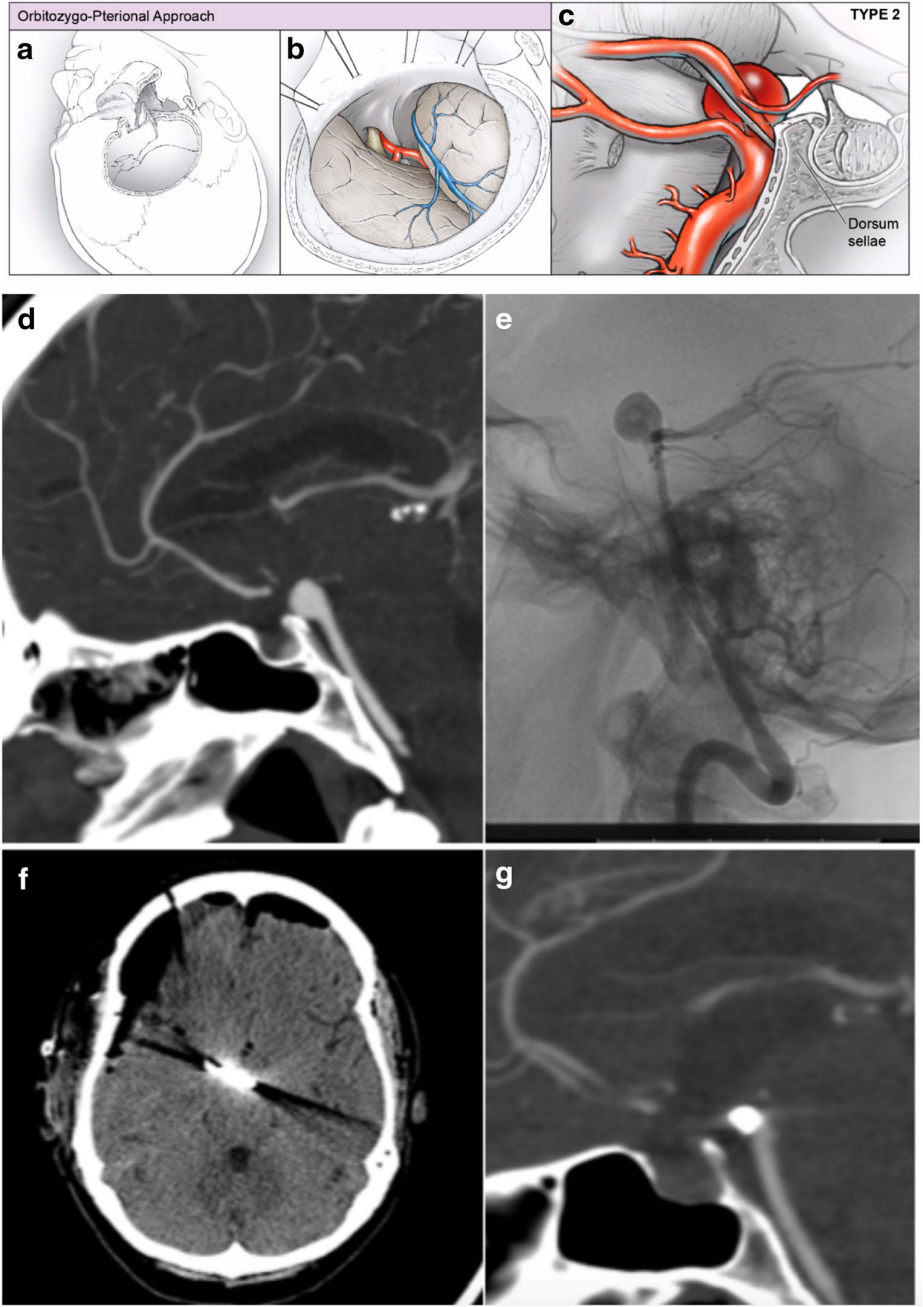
Representative orbitozygomatic-pterional approach to basilar artery aneurysms. (a–c) For basilar apex aneurysms located at or above the level of the dorsum sella, an orbitozygomatic-pterional approach offers visualization of the aneurysm neck. (a, b) After a standard curvilinear incision, a pterional craniotomy with orbitozygomatic osteotomies is performed. (c) Trans-Sylvian dissection is used to expose the basilar artery apex. The posterior communicating artery can be sectioned at the P1-P2 junction to aid in the medial mobilization of the ICA. (d–g) Representative images of management of a basilar apex aneurysm. (d, e) Preoperative sagittal CT angiogram and lateral angiograms demonstrate anterior and superiorly projecting aneurysm. (f) Immediate postoperative axial non-contrast CT scan and (g) 2-year follow-up CT angiogram demonstrate complete occlusion of the aneurysm
